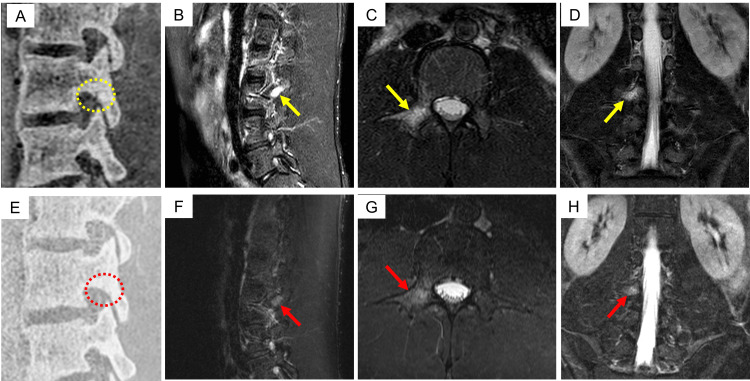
MRI images of Case 6 showing a lesion at the right L3 pars interarticularis. MRI scans obtained at the initial visit (A–D) and 1.5 months later (E–H) are presented. At the initial visit, a fracture line and bone marrow edema (BME) were observed (yellow circles and arrows). At the 1.5-month follow-up, the fracture line had disappeared, and BME was reduced (red circles and arrows). MRI, magnetic resonance imaging; BME, bone marrow edema; A, B, E, and F, sagittal view through the right L3 pedicle; C and G, axial view through L3; D and H, coronal view through the L3 pedicle.
